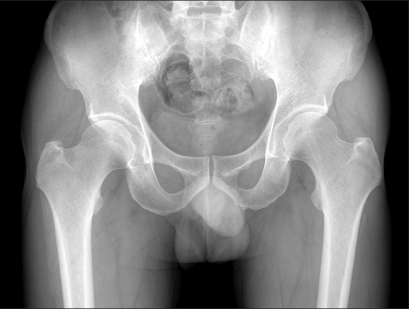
Both hip anteroposterior radiograph of a 46-year old male who suffered from left hip joint pain from 10 years ago shows a positive crossover sign in the right hip area.
